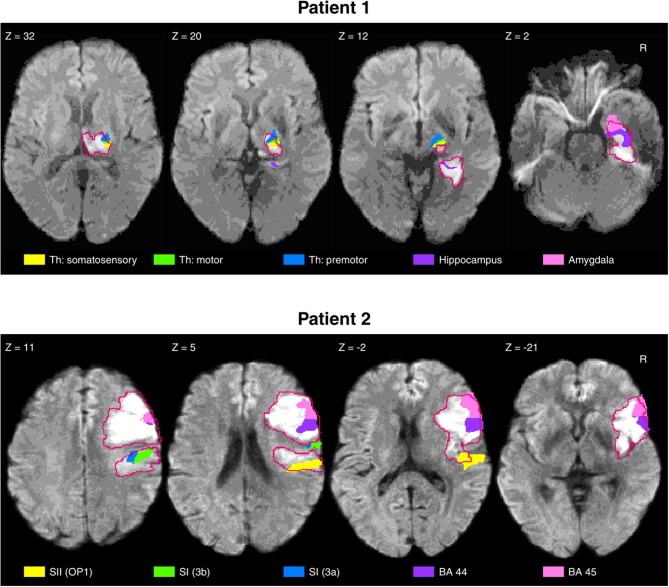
Structural MRI lesion analysis for patients 1 and 2. The lesion of patient 1 (Top) covered parts of the hippocampus and amygdala, but most importantly, regions of the thalamus with projections to sensorimotor regions. The lesion of patient 2 (Bottom) covered great extent of the Brodmann areas 44 and 45, and parts of the primary (SI) and secondary somatosensory cortices (SII), thus strongly affecting the sense of touch. The lesion borders (red outlines) were drawn on the original 3D images, using MRIcron software (21).
